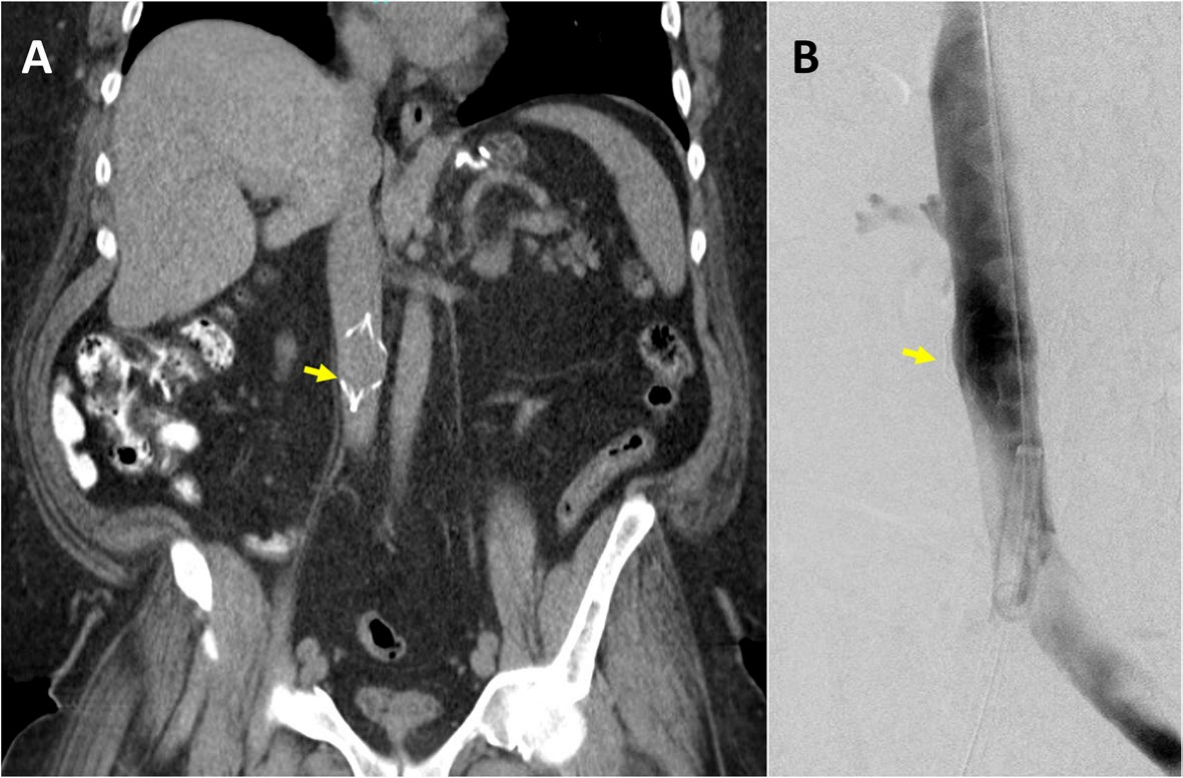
Pre-retrieval evaluation. A CT scan showed IVC filter (yellow arrow) embedded to the wall of IVC. B Initial injection through the pigtail catheter showed no thrombus within the IVC filter (yellow arrow)
